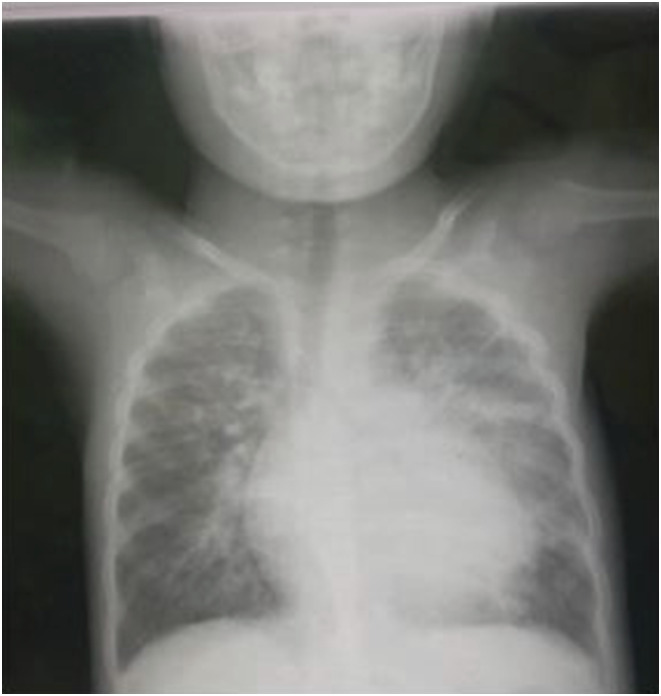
Chest X-ray of patient 3.
